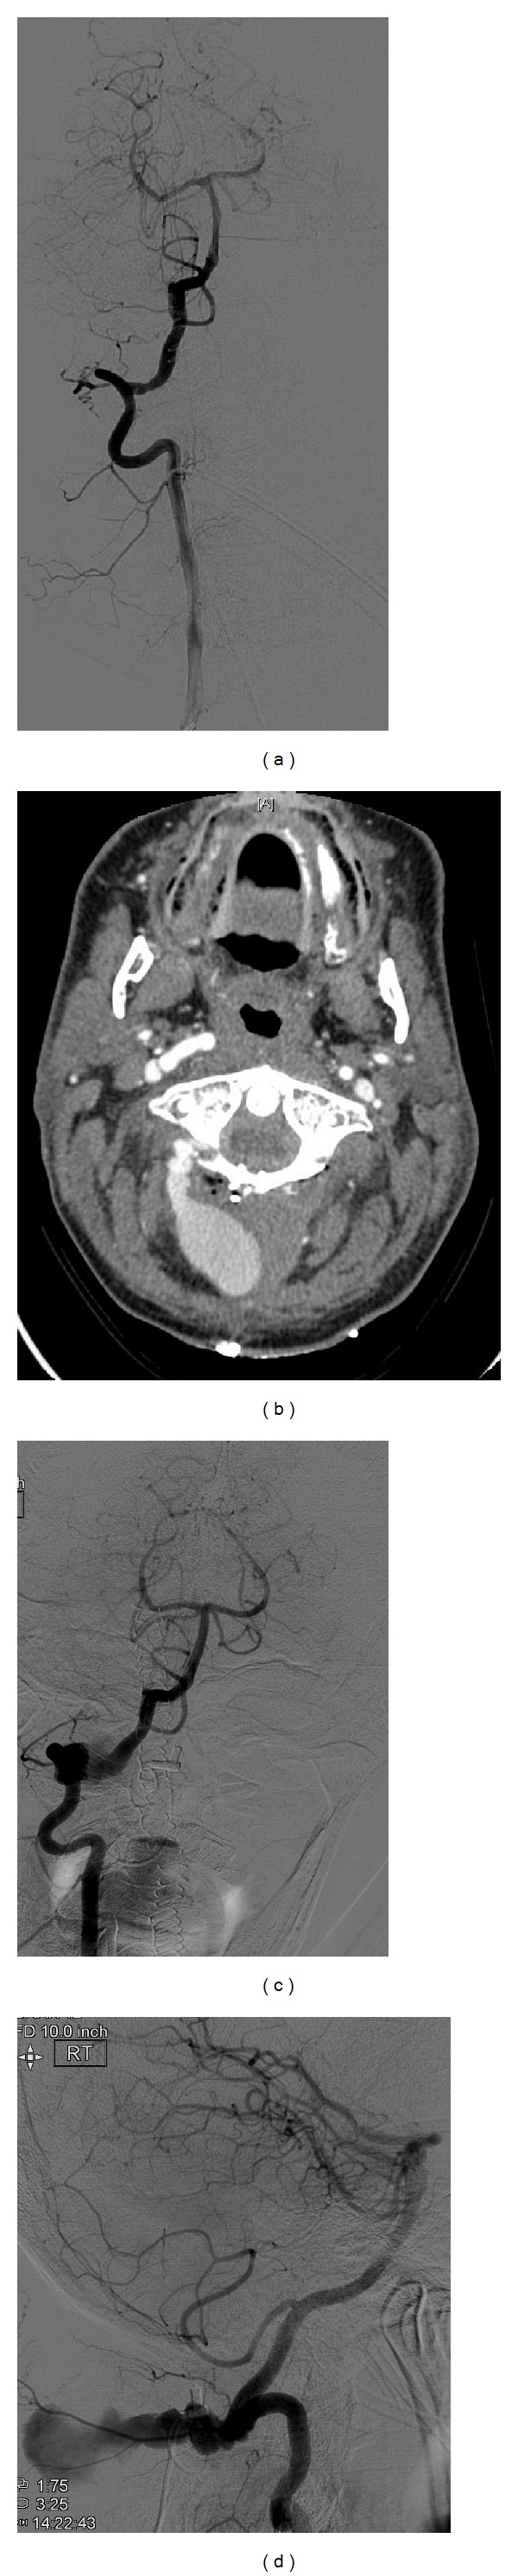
(a) shows normal filling of the right vertebral artery immediately following intraoperative injury: a small irregularity in the wall can be seen. (b) (CT angiogram) and (c) and (d) (digital subtraction angiogram) show a large saccular pseudoaneurysm arising from the V3 segment of the vertebral artery. The imaging was performed on the third postoperative day.
